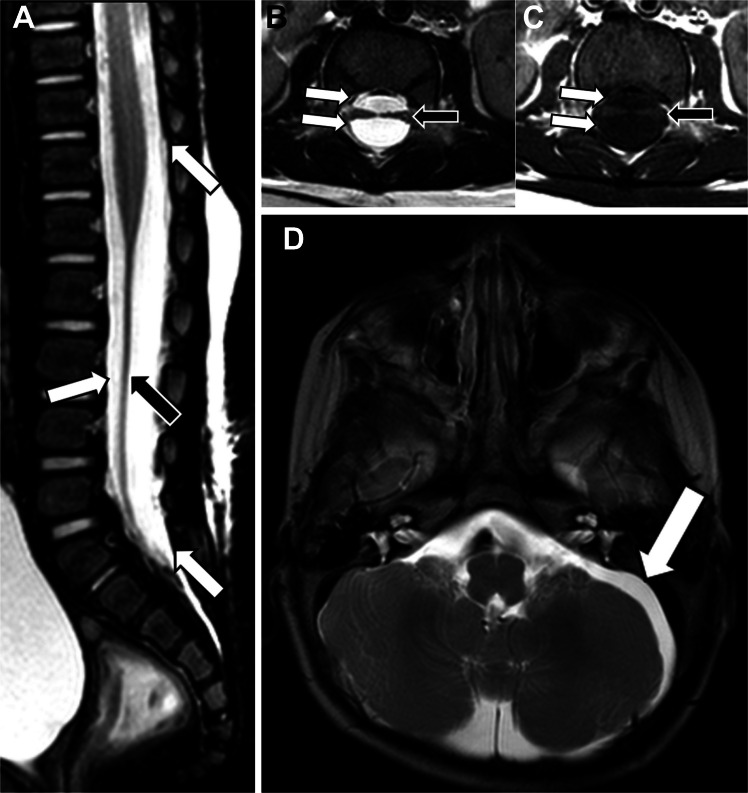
An 8-month-old male with suspected abusive head trauma and a thoracolumbar CSF-isointense extra-axial collection. Sagittal T2-weighted (A), axial T2-weighted (B), and T1-weighted (C) MR images of the thoracolumbar spine demonstrate a CSF-isointense dorsal and ventral fluid collection (*white arrows*) extending from T11 to the distal thecal sac, with compressed cauda equina nerve roots (*black arrows*). Axial T2-weighted image of the brain (D) demonstrates a CSF-isointense subdural collection in the left posterior fossa (*arrow*). No abnormality was seen in the cervical spine again highlighting the importance of whole-spine imaging (not shown)
